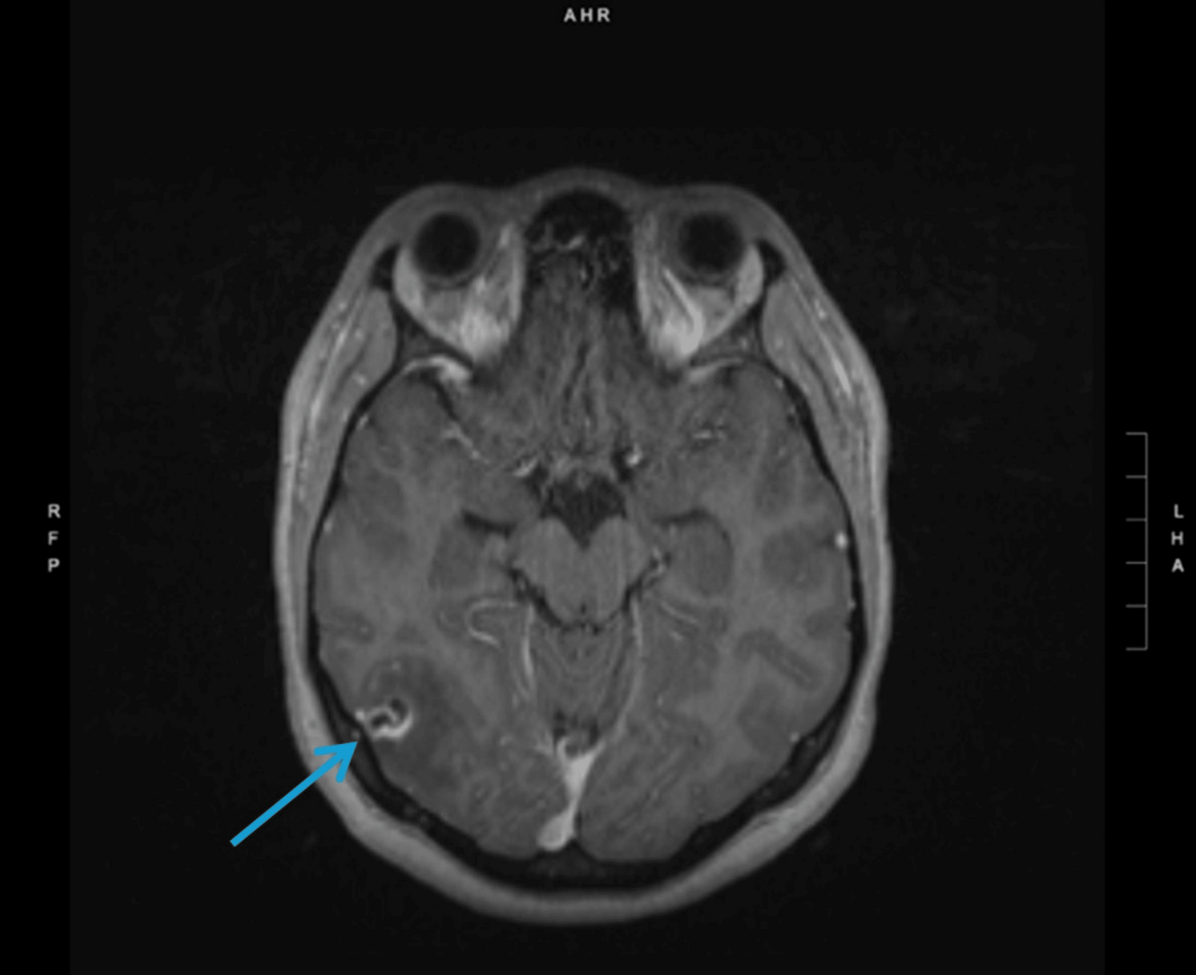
MRI showing a 12 mm ring-enhancing lesion with a central spot situated in the right lateral occipital lobe with surrounding edema
